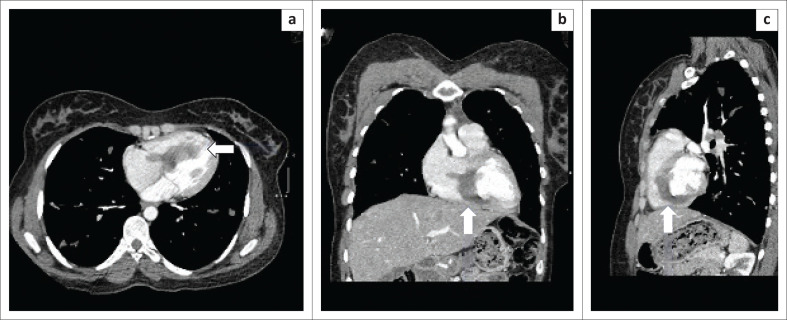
Computed tomography of the chest: (a) axial; (b) coronal; (c) sagittal demonstrating a well-defined interventricular hypodensity protruding into the right ventricle and right atrium (arrows).
